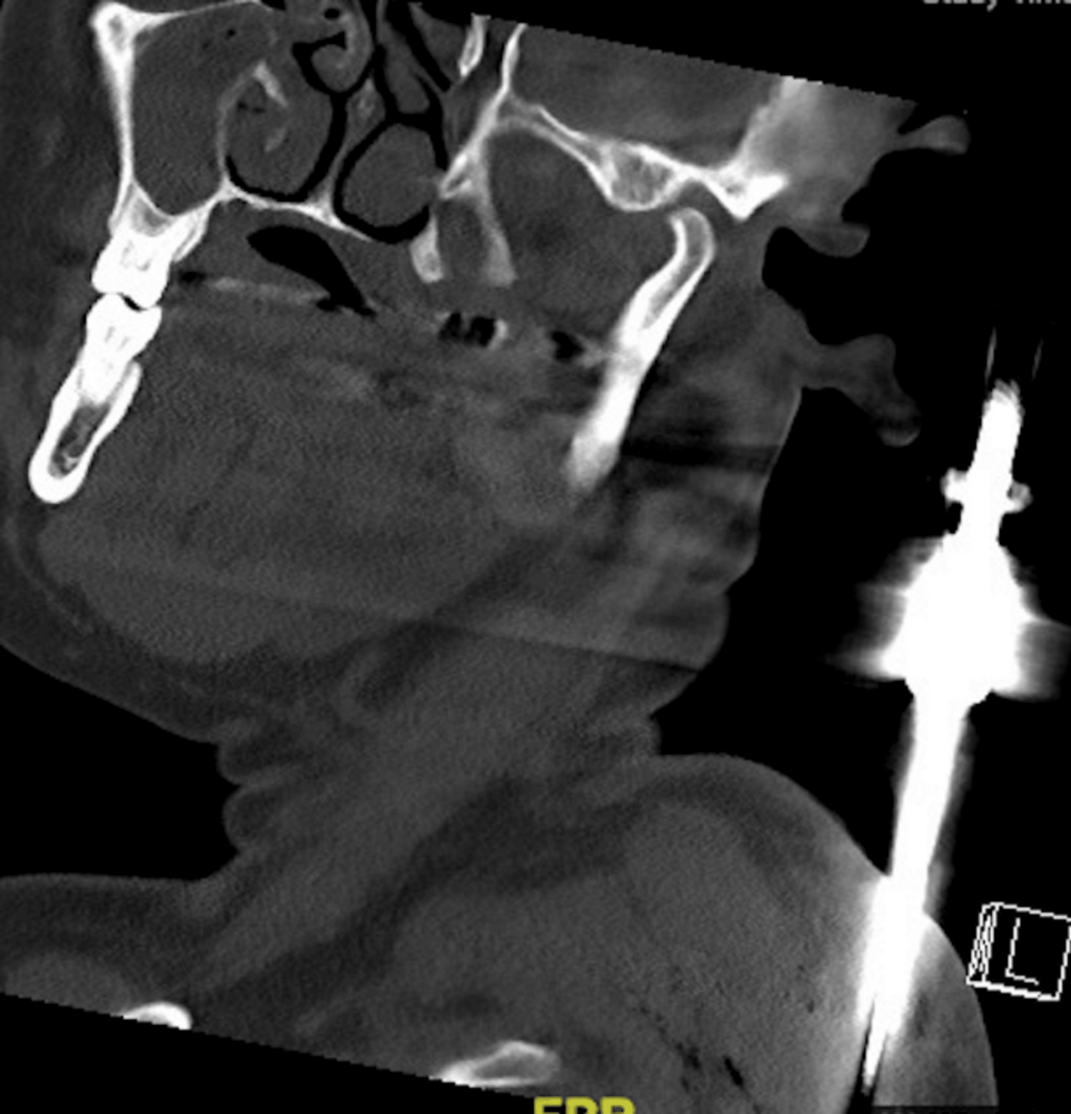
A CT scan of the thorax showing the knife lying along the medial border of the scapula.
